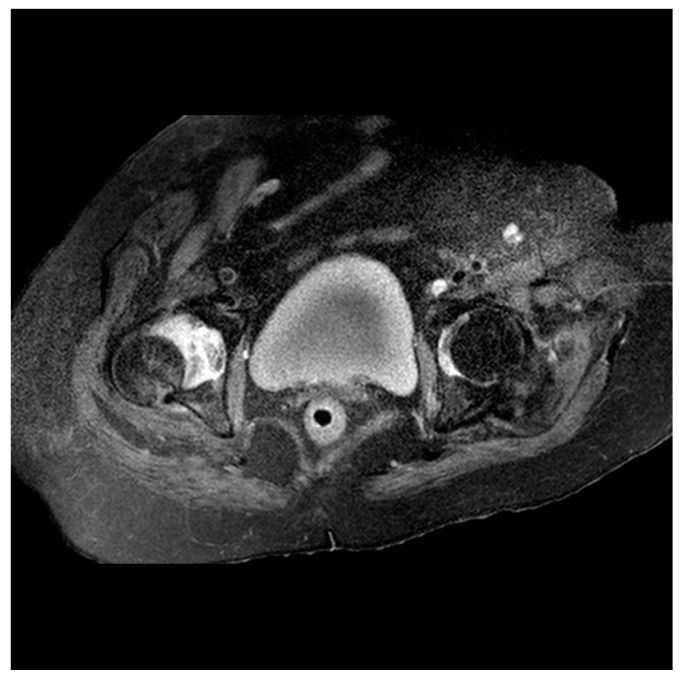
Pelvic Magnetic Resonance Imaging (MRI). The viability of the femoral head and the absence of fractures are confirmed.
